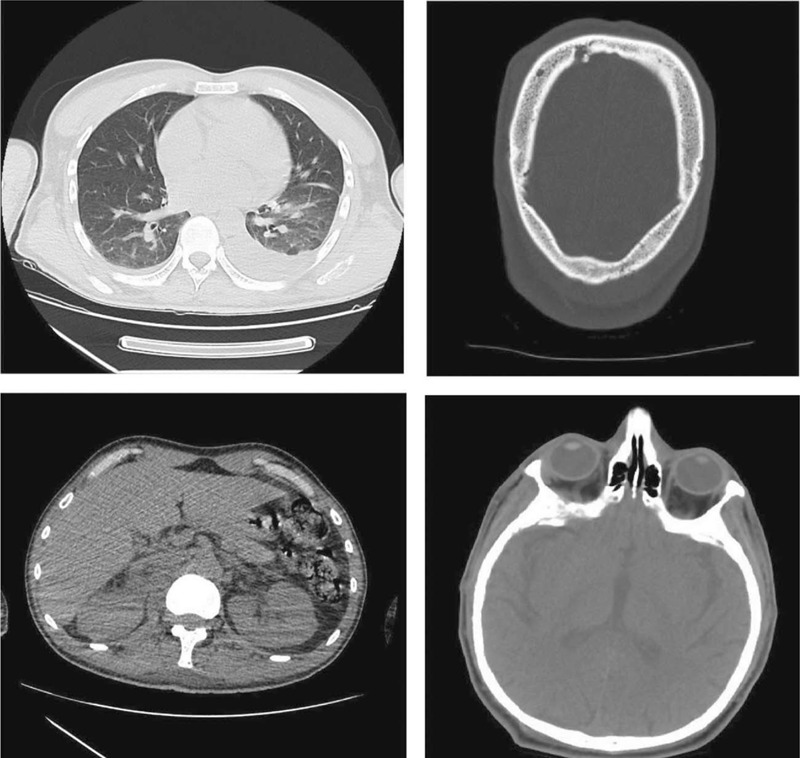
CT scan sections. CT = computed tomography.
